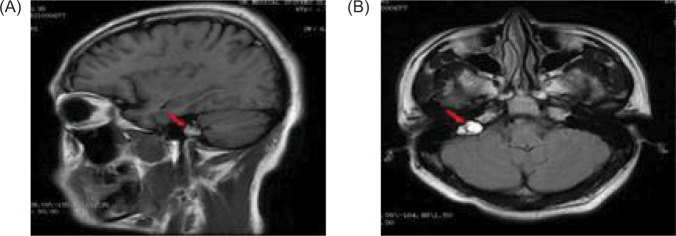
A magnetic resonance imaging (MRI) of the cranial reveals a possible mass in the right temporal bone jugular foramen, with a cross-sectional area of approximately 12×18 mm (red arrow); (A) sagittal position, and (B) flair view.
